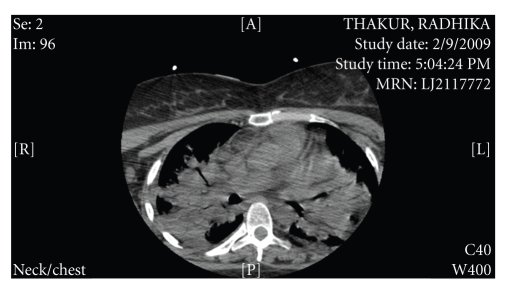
CT chest showing the diffuse bilateral pulmonary infiltrates.
